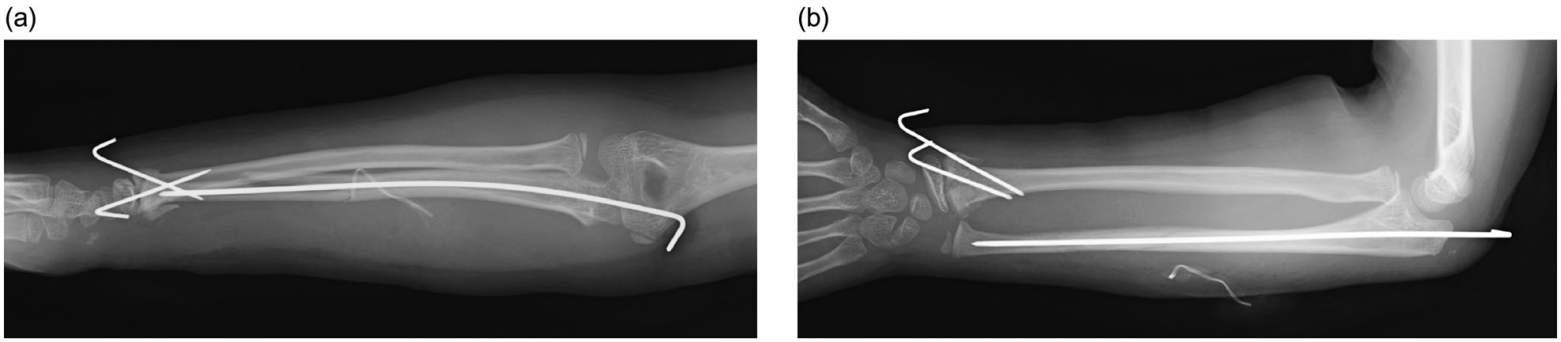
Anteroposterior (a) and lateral (b) views of radiographs at immediately after surgery.
